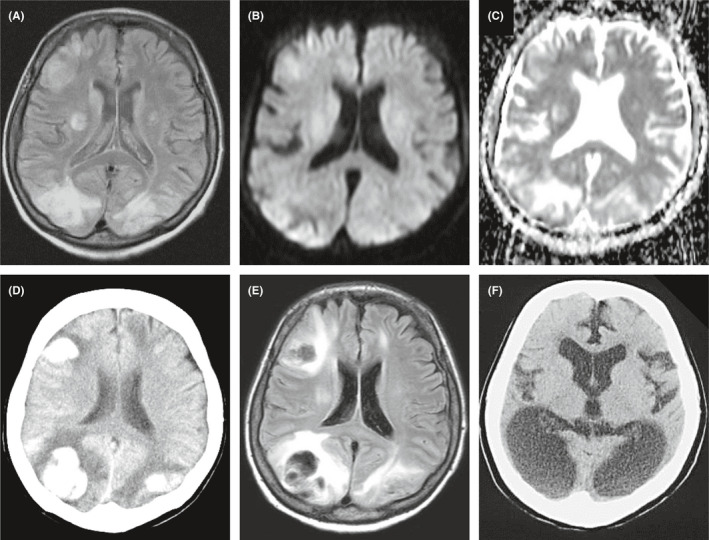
Head MRI and CT of Case 1. MRI on admission shows high‐intensity lesions in FLAIR, DWI, and ADC (A–C). Follow‐up CT and MRI show multiple intracranial hemorrhages (D, E). One‐year follow‐up CT showing occipital lobe‐dominant brain atrophy (F)
